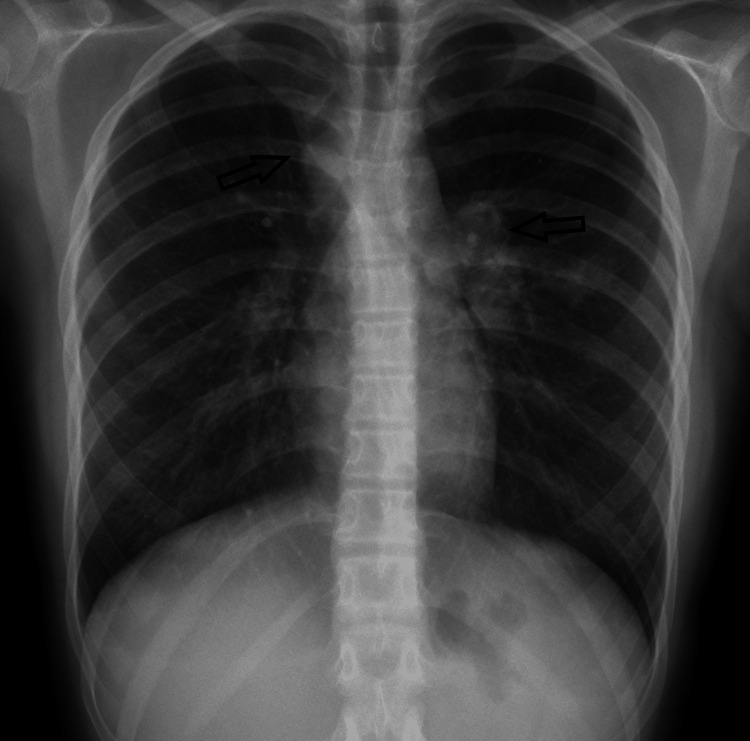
Chest x-ray revealed right superior mediastinal opacity in the right paratracheal stripe. Prominent left hilar and left parenchymal non-homogeneous opacity pointed at with the arrows.
